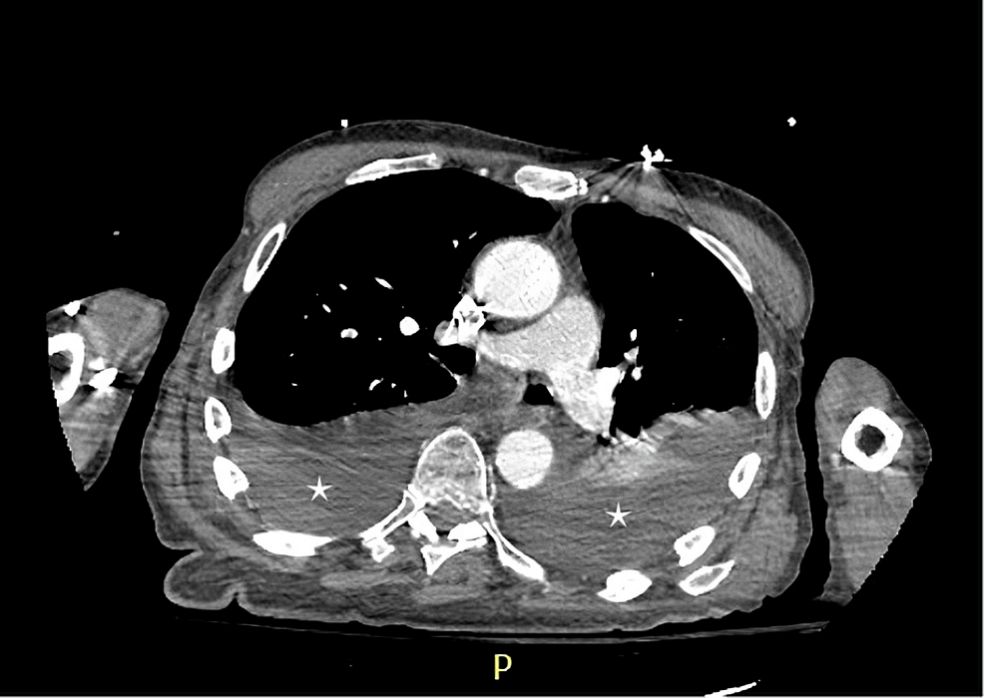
Computed tomography scan of the lungs showing bilateral pleural effusions (marked with *).
